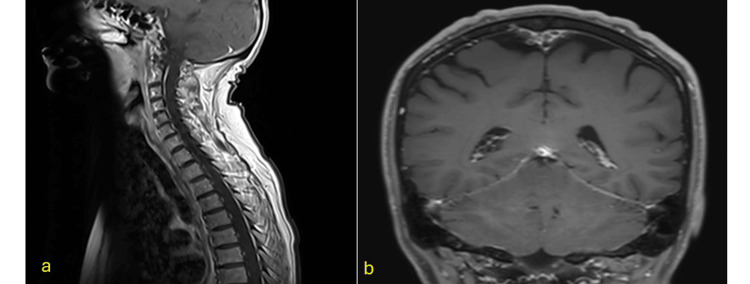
Imaging follow-up MRI follow-up at six months showed regression of intramedullary lesions (a). Contrast enhancement of cerebellar leptomeninges had also disappeared (b).
